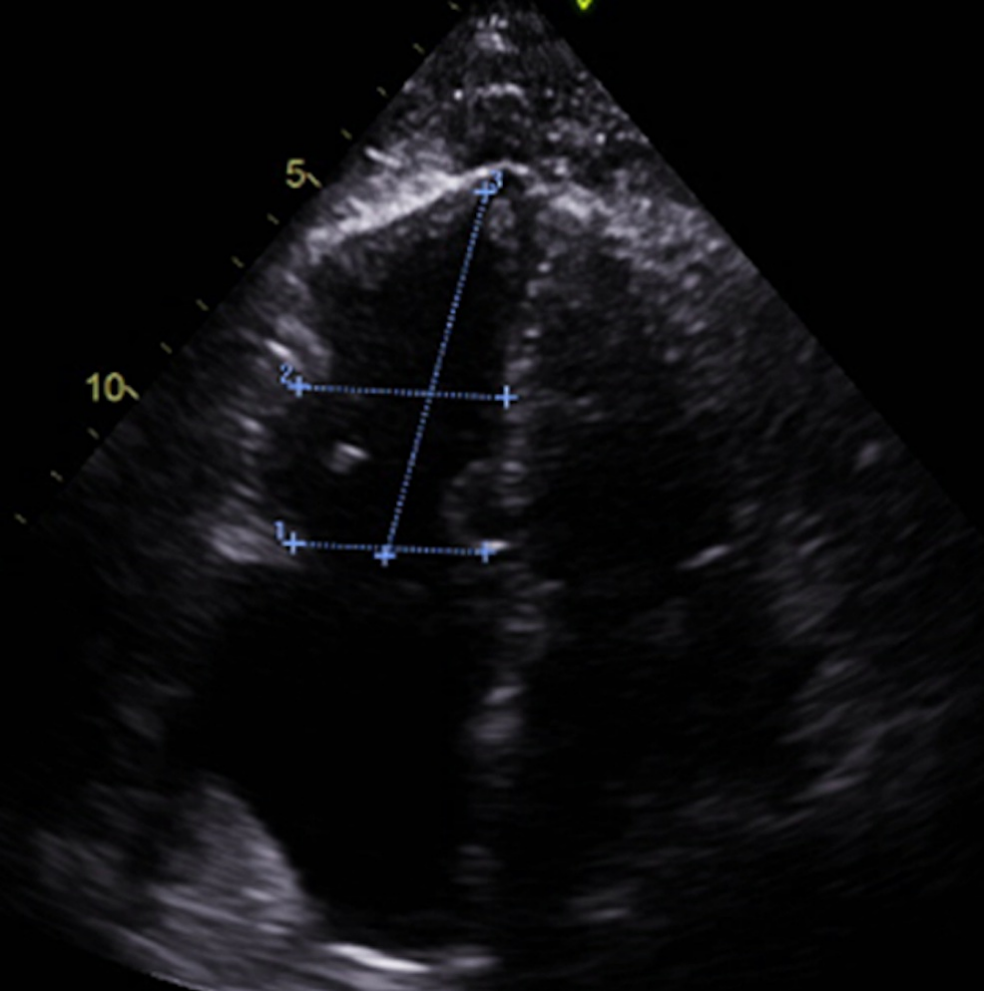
Two-dimensional echocardiogram showing RV dilation. A4CH RV diameter was 3.7 cm suggestive of RV dysfunction. In comparison, the A4CH LV diameter was 3.1 cm. RV: right ventricle, A4CH: apical 4 chambers, LV: left ventricle.
